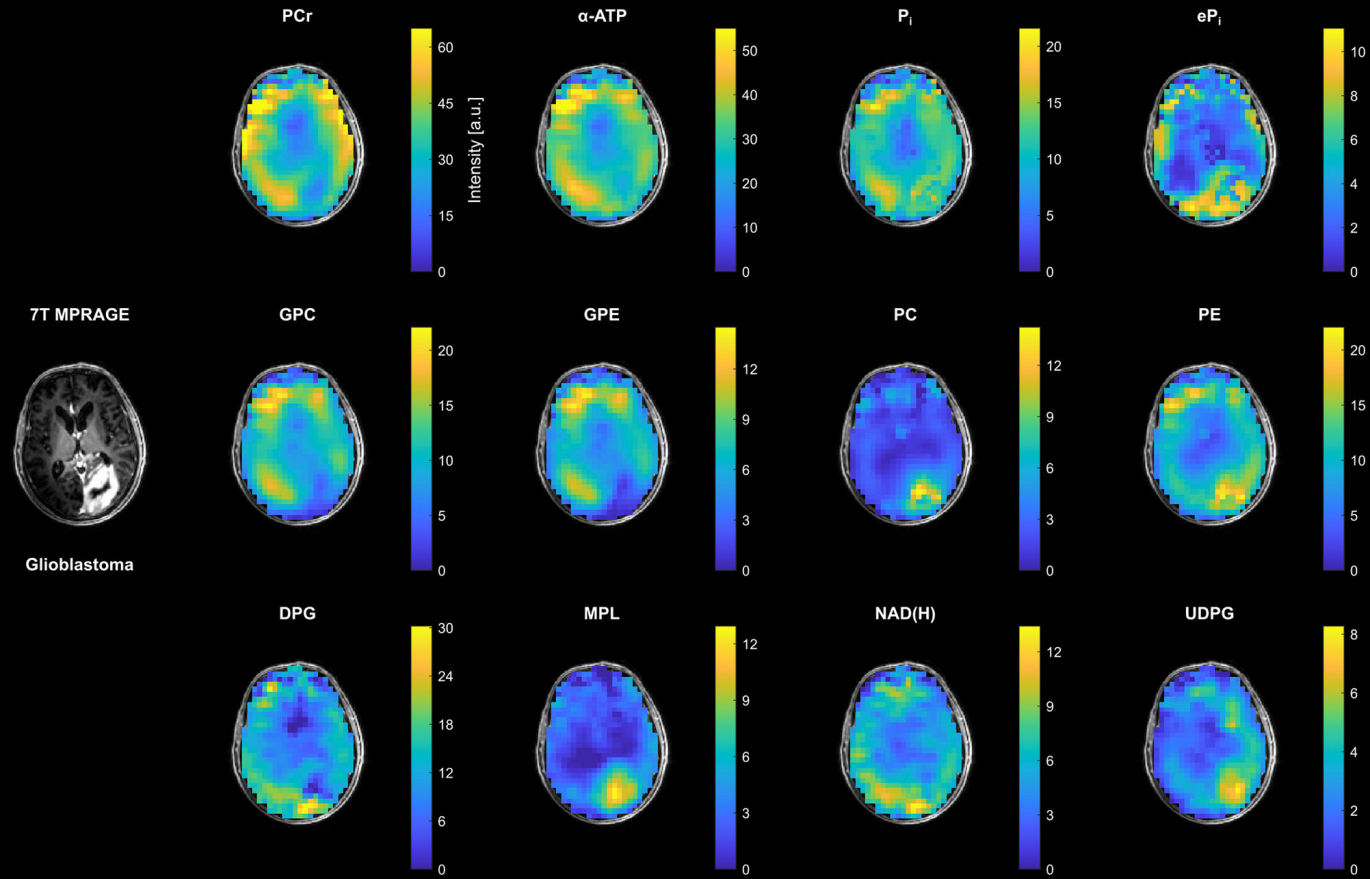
Representative transversal slices of all quantified 3D metabolite maps from the patient with high-grade glioma (glioblastoma; cf. Figure 1C), shown as intensities in arbitrary units. Note the different scales for the individual metabolites, and that all maps are subject to the coil sensitivity profile. For visual guidance, the metabolite maps are masked to show only brain parenchyma as displayed on the 7T non-enhanced T1-weighted MPRAGE images.
